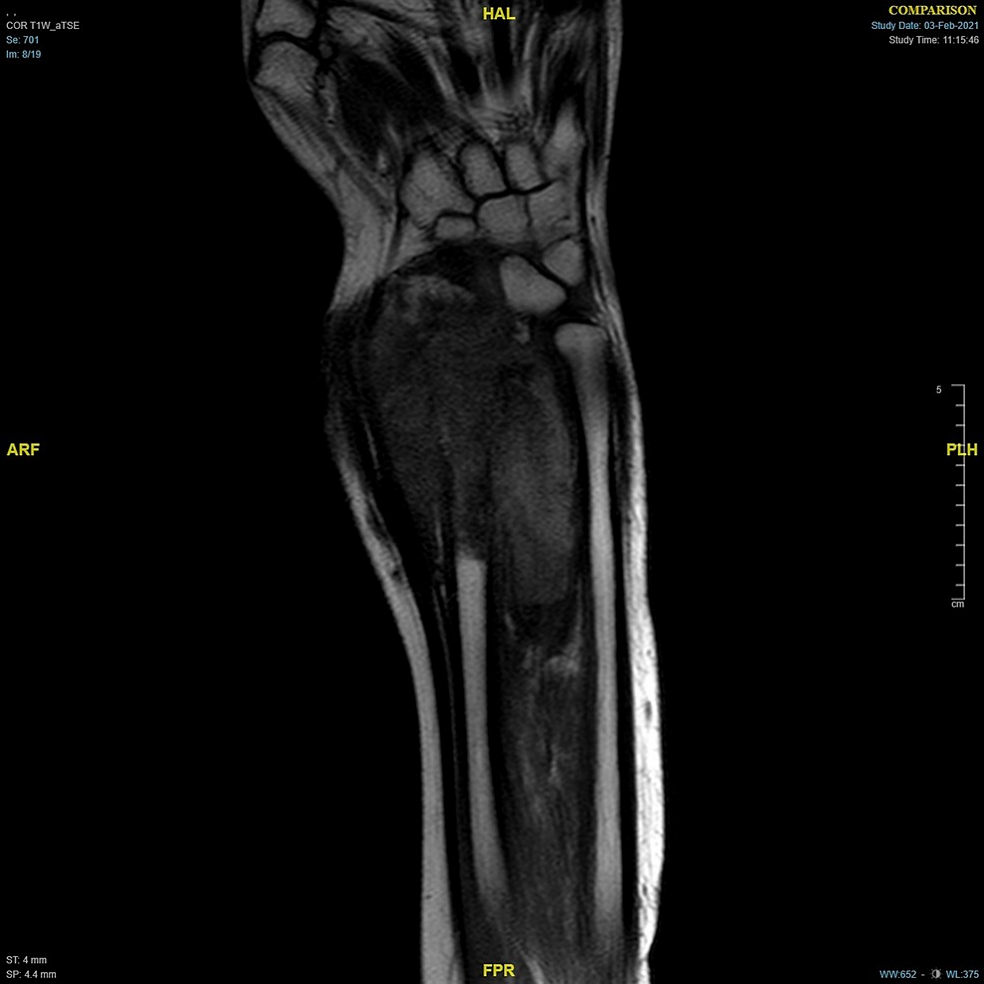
MRI of the right upper limb shows soft tissue mass occupying the distal right radius and ulna with extension to the carpal bones.
